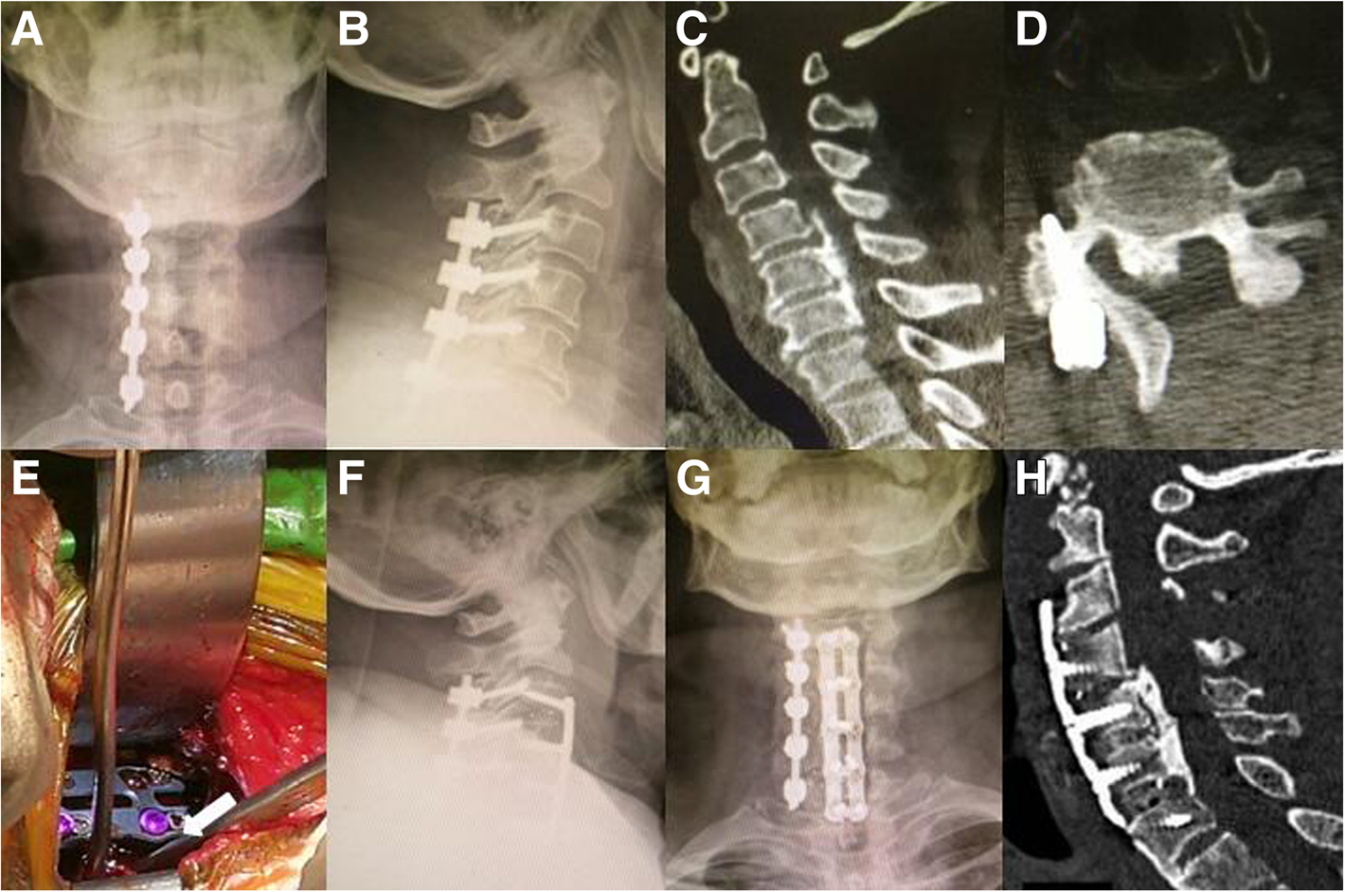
A revision case of a 61-year-old man 12 months after initial posterior laminectomy. a, b The anterior–posterior and lateral images showed the loss of cervical lordosis after the initial laminectomy. c, d The CT scan showed that there was a continuous type of OPLL, and only a semilaminectomy with one-sided lateral mass fixation was done in the initial surgery. e The intraoperative photo showed that after installation of the intervertebral cages and anterior cervical plate, we used 1-mm Kerrison rongeurs to remove the posterior vertebral wall on the bottom of the troughs for isolation of the VOC (arrows). f, g The postoperative anterior–posterior and lateral images showed good internal fixation position and improved cervical lordosis. h The CT scan showed that the VOC was hoisted forward, and the cervical spinal canal was obviously wider than it was before
